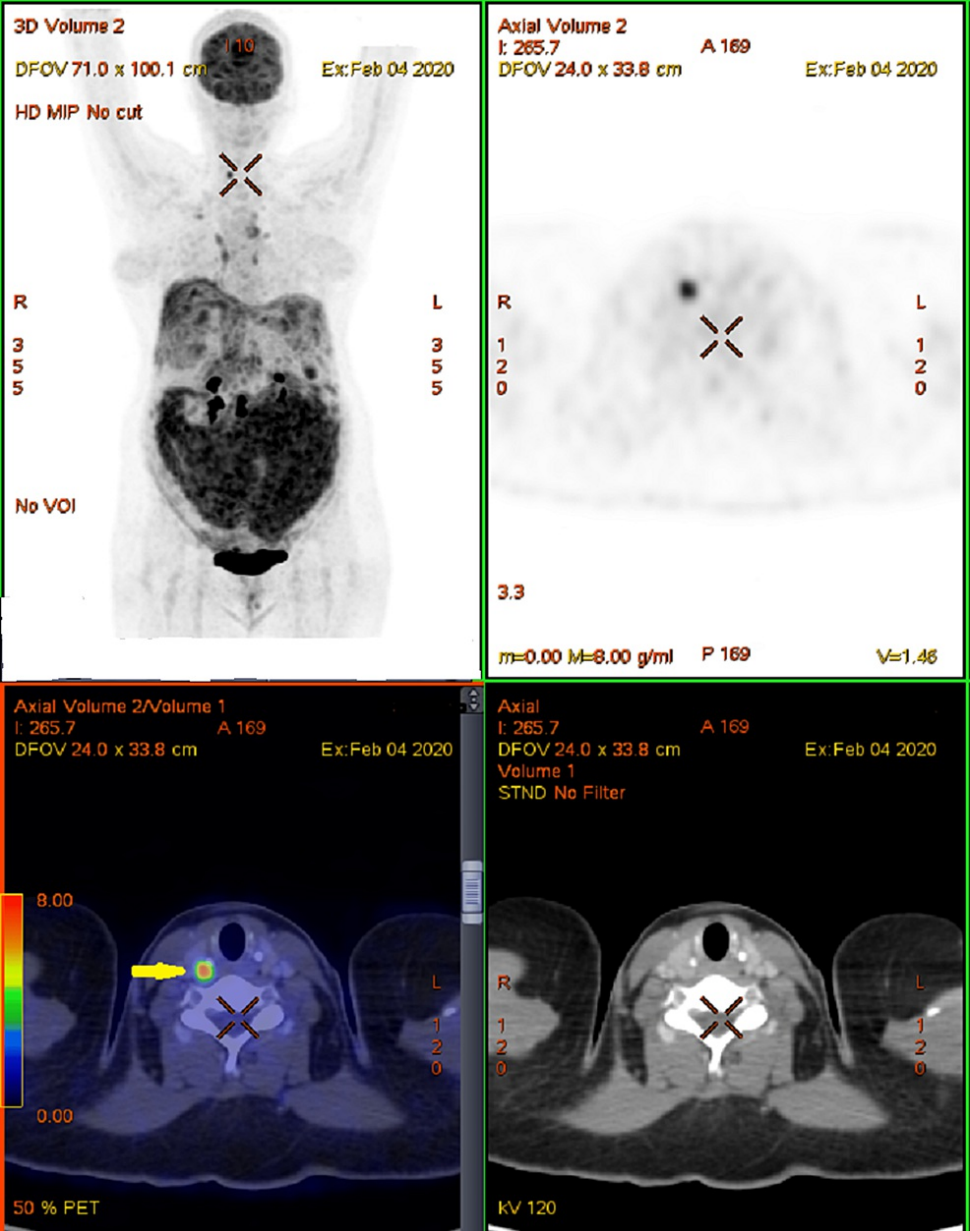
Pretreatment PET-CT images The images are showing supra and infra-diaphragmatic lymph nodes and focal uptake in the right thyroid (yellow arrow). PET-CT: positron emission tomography-computed tomography
